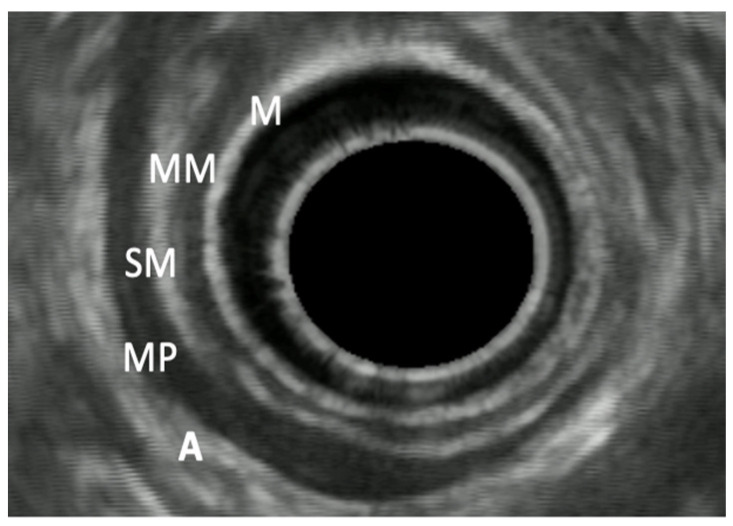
Endosonographic image revealing the five layers of the esophageal wall: mucosa (M), muscularis mucosa (MM), submucosa (SM), muscularis propria (MP), and adventitia (A).
